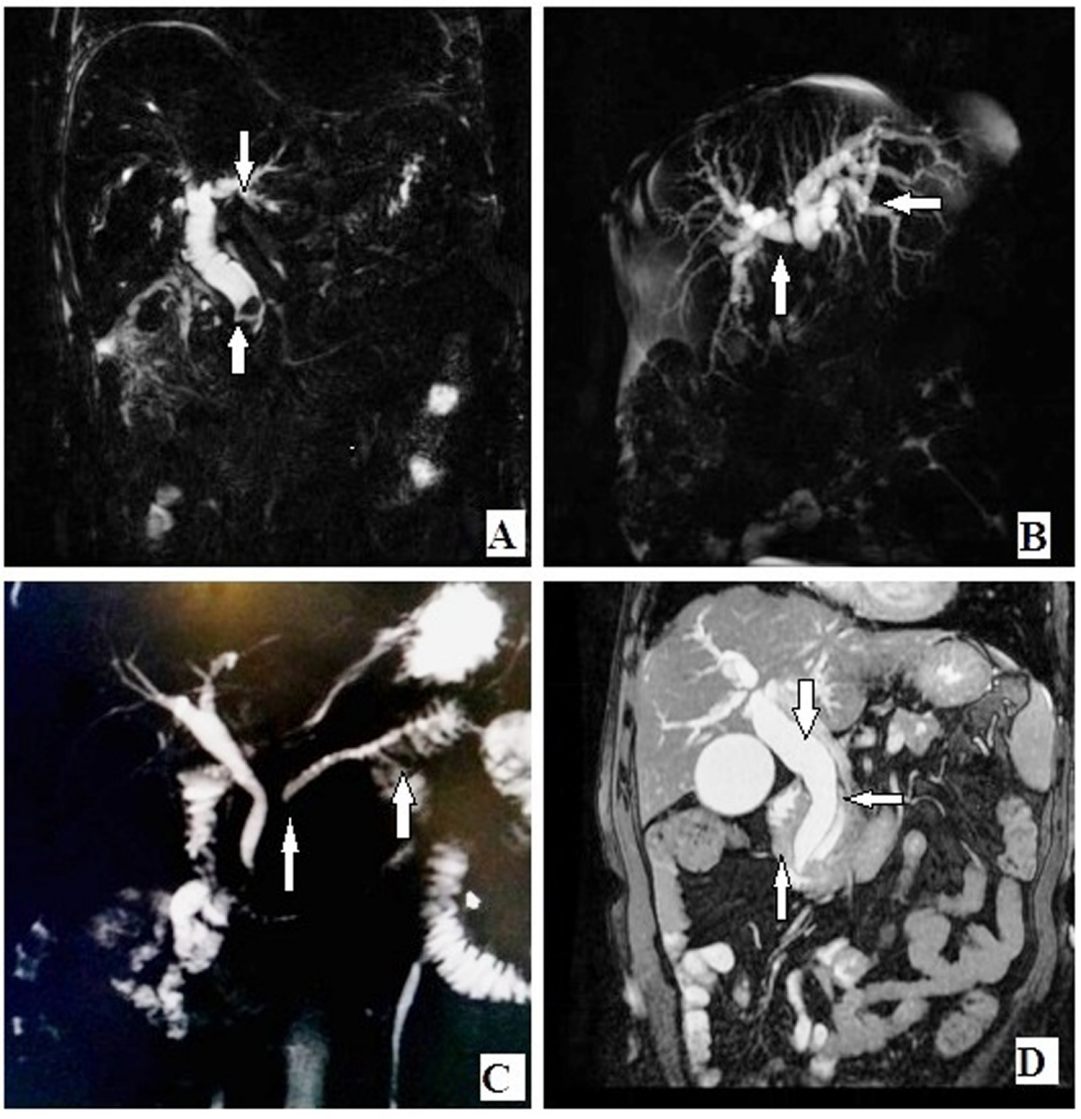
(A) Coronal MIP reformat showing CBD calculus at distal end with proximal obstructive bile duct dilatation. (B) Coronal MIP reformat showing hilar mass lesion with proximal bile duct dilatation and non-confluence of right and left hepatic ducts. (C) Coronal MIP reformat showing stricture of MPD with upstream dilatation in a case of chronic pancreatitis. (D) Coronal T2-weighted fat-saturated image showing neoplastic mass of periampullary region with stricture of distal CBD and distal MPD and dilatation of proximal pancreatic duct and proximal CBD and IHBR. CBD, common bile duct; MIP, maximum intensity projection; MPD, Main pancreatic duct; IHBR, Intrahepatic biliary radicals.
